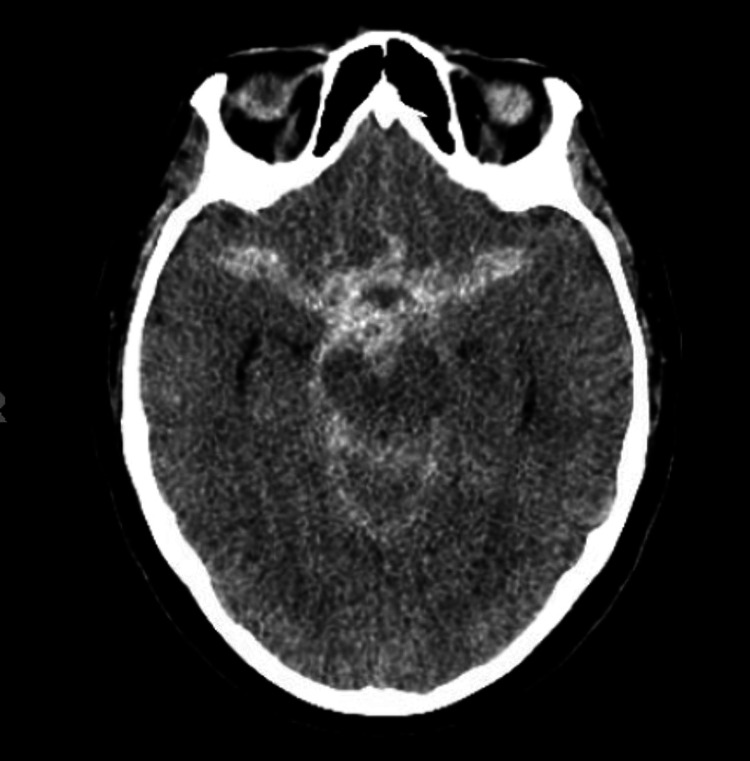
Non-contrast computed tomography scan with a diffuse cisternal subarachnoid hemorrhage
